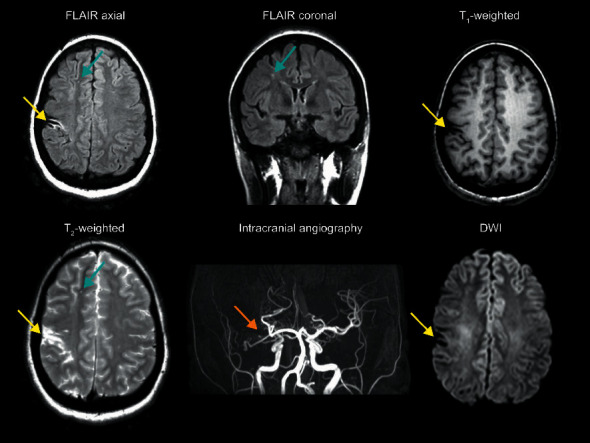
Representative scans from the multimodal magnetic resonance imaging protocol from a Black 9-year-old female with hemoglobin SS phenotype. Chronic stroke is apparent (yellow arrows), with additional right parietal and frontal lobe chronic silent cerebral infarcts (blue arrows), which largely colocalize with patchy T_2_-hyperintense foci. On intracranial angiography, there is near-complete occlusion of the terminus of the right internal carotid artery with a very short proximal portion of the right M1 segment visible (orange arrow). Extensive collateral vessels are identified extending into the basal ganglia region on the right side. No evidence of acute infarct is visible on diffusion-weighted imaging (DWI).
